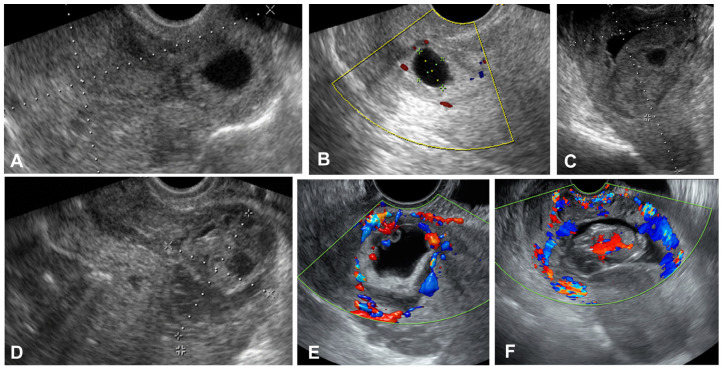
Ultrasonographic images of cesarean scar pregnancy. (A) Low implantation and bulging of gestational sac in lower uterine segment. (B) Low implantation of small blood flow around the gestational sac with empty uterine cavity and cervical canal. (C,D) Heterogenous mixed placental tissue bulging in lower uterine segment. (E,F) Color Doppler imaging showing blood flow around the gestational sac at the site of implantation suggestive of “hypervascularity”.
